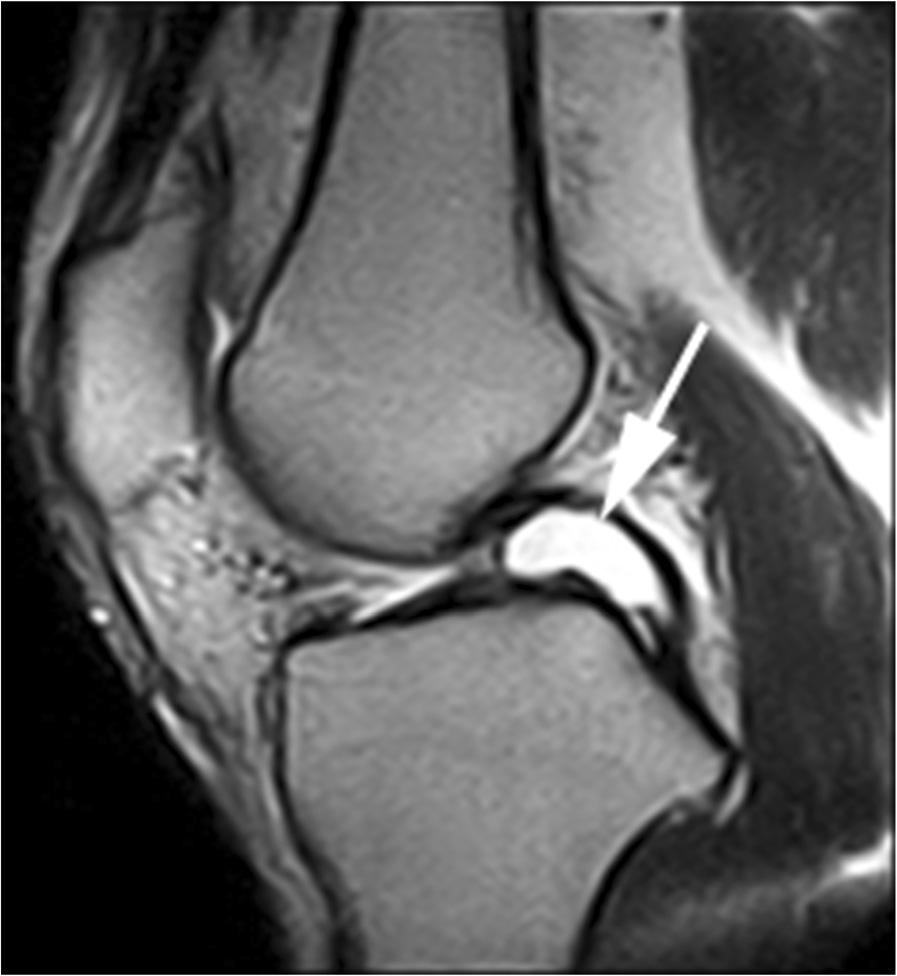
Cyst of the PCL in the sagittal plane on T2-weighted images.
